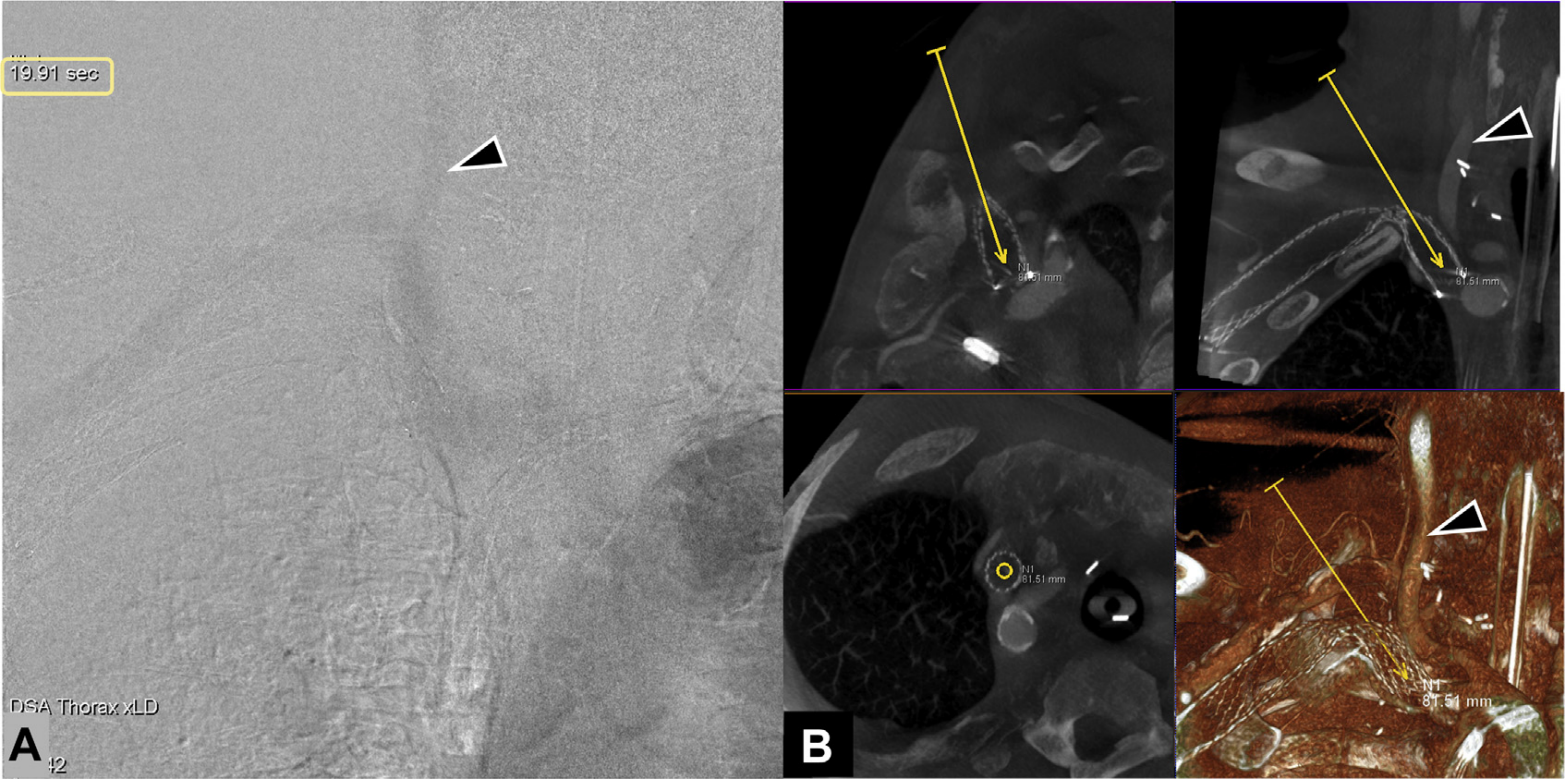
Timing Bolus Digital Subtraction Angiography and Intraoperative Cone-Beam Computed Tomography Venography Planning (A) Timing bolus digital subtraction angiography was performed to derive the time to visualize arterial structures such as the right subclavian artery and the right common carotid artery (arrowhead) before cone-beam computed tomography angiography. (B) A virtual needle path (N1) was planned (yellow line) in 3-dimensional multiplanar and volume-rendered reconstructions of cone-beam computed tomography images. The yellow circle in the axial view denotes the target site inside the occluded stent. The arrowhead denotes the right common carotid artery. N1 = needle path 1.
